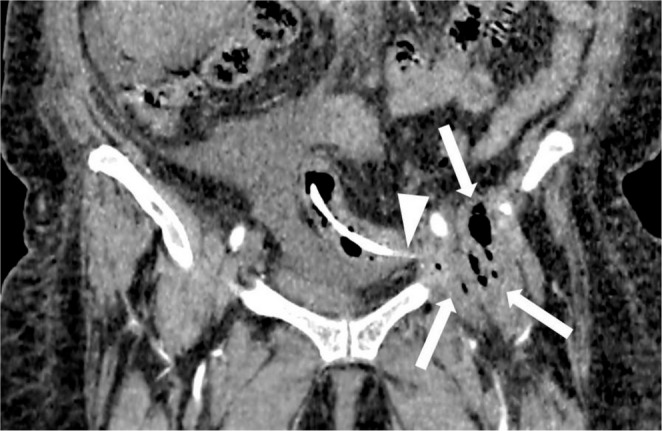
Coronal contrast-enhanced CT of the pelvis in soft tissue window. A biliary stent perforating the sigmoid colon wall (arrowhead) is seen. At the level of the migratory stent, a 38 × 25 mm abscess with a thick wall within the iliopsoas muscle, containing multiple foci of air and demonstrating peripheral contrast enhancement, is observed (arrows). The abscess is extending inferiorly along the iliopsoas bursa
